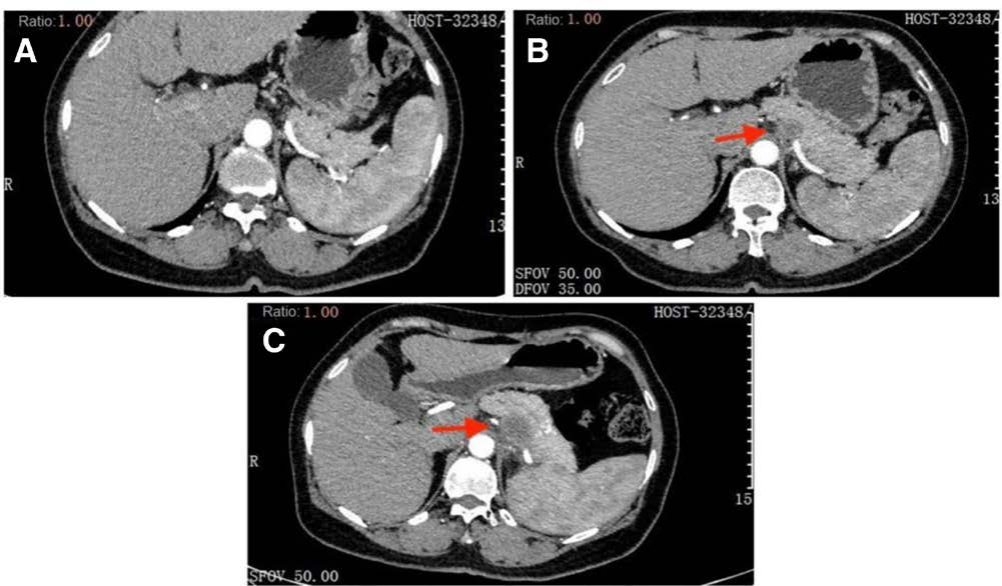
Abdominal CT images at different stages. The major lesion (red arrow) is at the pancreatic body. (A) Abdominal CT image on October 5, 2020 showed no space-occupying lesion in the pancreas. (B) Examinations showed persistent remission of the pulmonary lesion during immunotherapy, but the abdominal CT scanning on March 9, 2021 showed a space-occupying lesion in the pancreas. (C) CT scanning on June 9, 2021 showed persistent remission of the pulmonary lesion during the immunotherapy, but the pancreatic lesion enlarged. CT = computed tomography.
